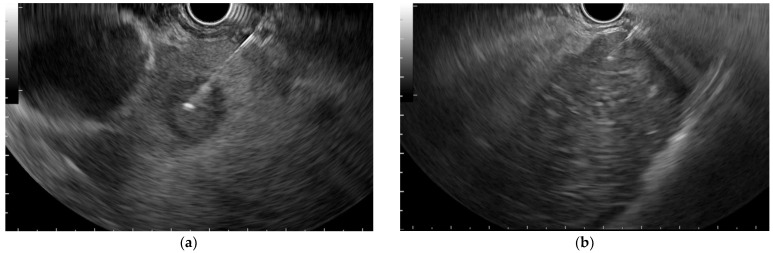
Endoscopic ultrasound tissue acquisition. (a) Hepatic metastasis of pancreatic adenocarcinoma; (b) hepatocarcinoma.
